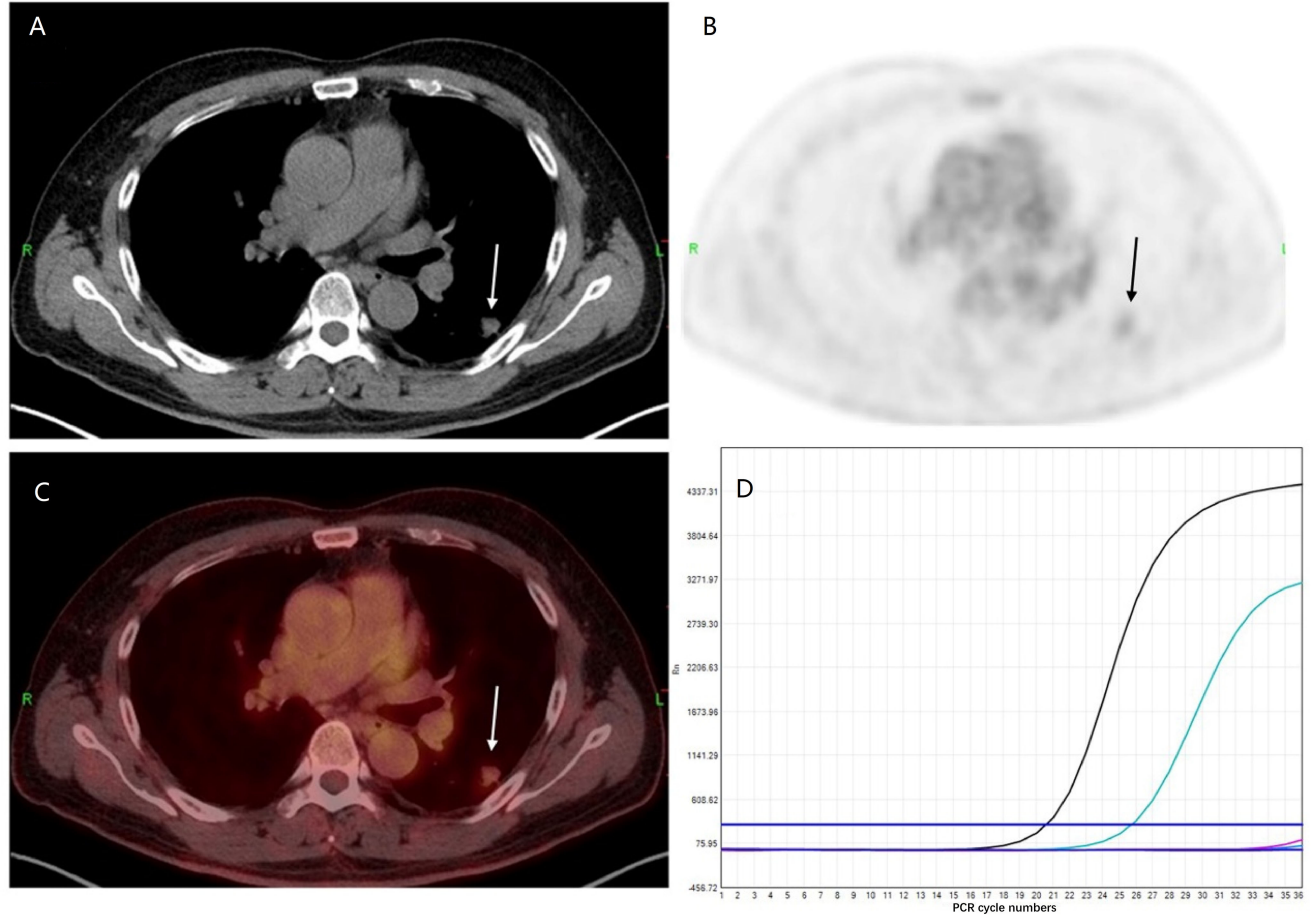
Representative epidermal growth factor receptor (EGFR) status and ^18^F-FDG PET/CT finding. A 53-year-old man with EGFR wild-type lung adenocarcinoma. (A) CT, (B) PET, and (C) PET/CT fusion images show a 1.0-cm-sized mild ^18^F-FDG uptake mass in the dorsal segment of the left lower lobe (SUVmax = 2.3) (arrow). (D) Genetic testing demonstrates wild-type EGFR status.
